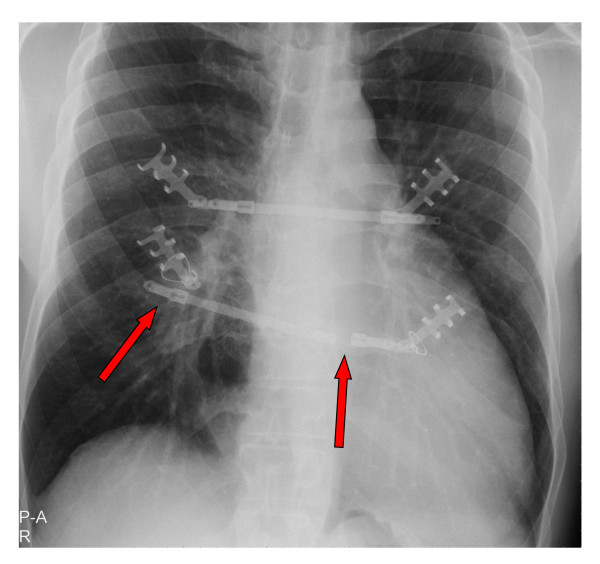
X-ray at readmission. Red Arrows indicating the crack and dislocation of the metal bars.
